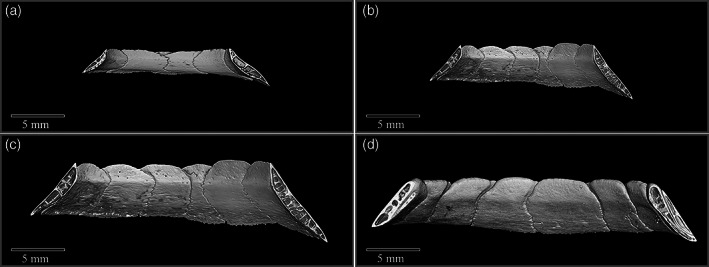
Midline cut away micro‐CT image to show the curvature of the inner and outer surfaces of the scleral ring in two little penguins (a and b, likely to be *Eudyptula novaehollandiae*, AV 958A and AV 989A), one Snares penguin (*Eudyptes robustus*, AV 1181A) and one king penguin (*Aptenodytes patagonicus*, K3). The outer surface appeared almost flat but the inner was concave inwards. The curvature of the scleral ring did vary but, excluding size differences, without digital analysis there did not appear to be greater variation in shape between species and genera than between individuals of the same species, as demonstrated by the obvious curvature difference between (a) and (b), both *Eudyptula*, but not between (b) and (c)
